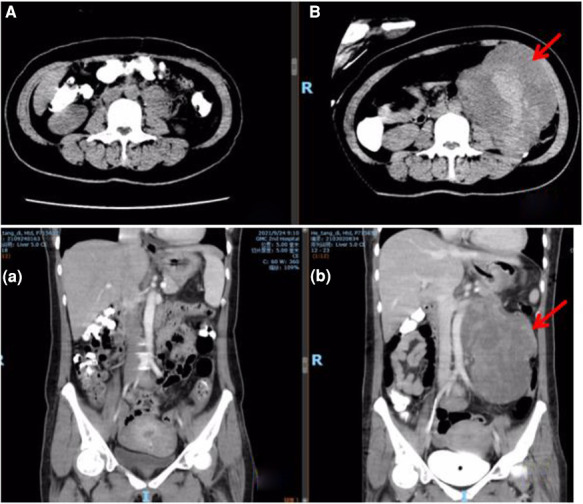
Abdominal CT comparison. (A/a) postoperative CT at five months; (B/b) preoperative CT (arrow refers to the tumor).
